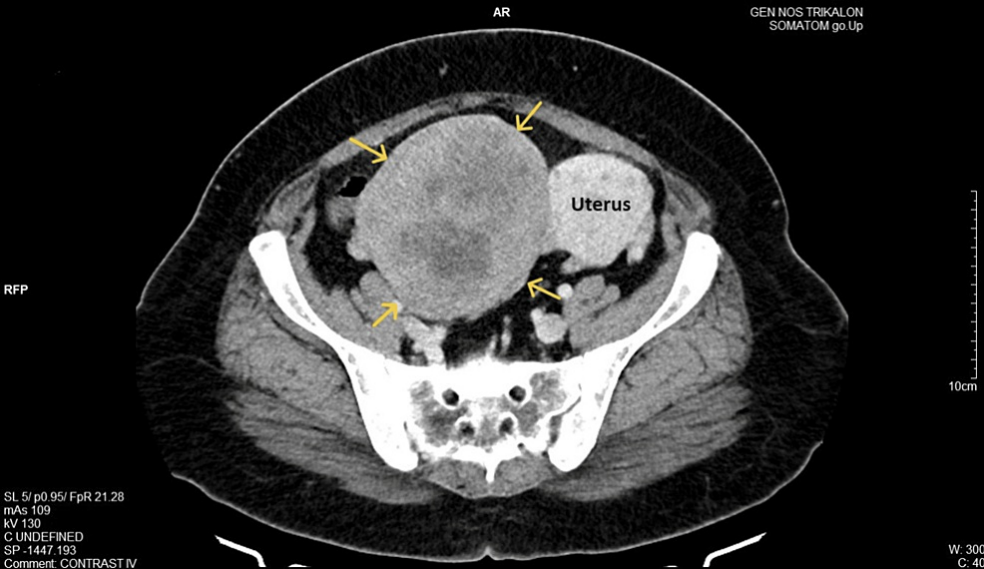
CT imaging of primary broad ligament fibroid The image depicts the presence of a large exophytic mass (yellow arrows), which appears to be attached to the right lateral wall of the uterus and occupies a substantial part of the peritoneal cavity
